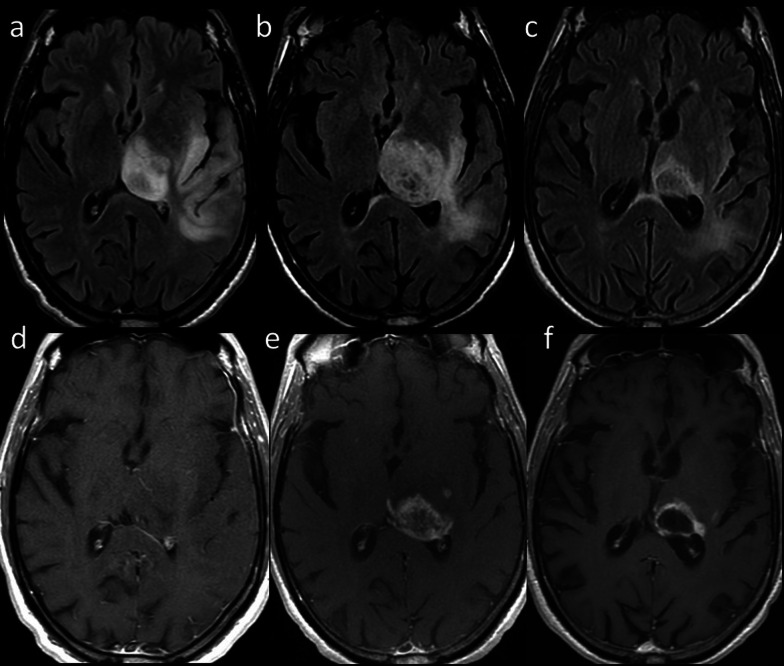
Pseudoprogression. 71-year-old man with glioblastoma treated with ChT and RT. Axial FLAIR (upper row) and contrast-enhanced T1-weighted (lower row) images before (a, d), during (b, e), and 3 months after treatment (c, f) showed initial occurrence of increasing size and enhancement of the lesion, followed by reduction of both signal changes and enhancement at the 3-month follow-up
